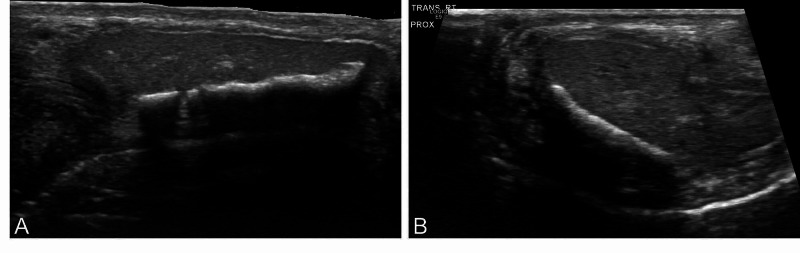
Penile Doppler ultrasound imaging demonstrating a 2.5-cm dorsal density with acoustic shadowing A) Longitudinal view of the right corpora. B) Transverse view primarily of the right corpora
